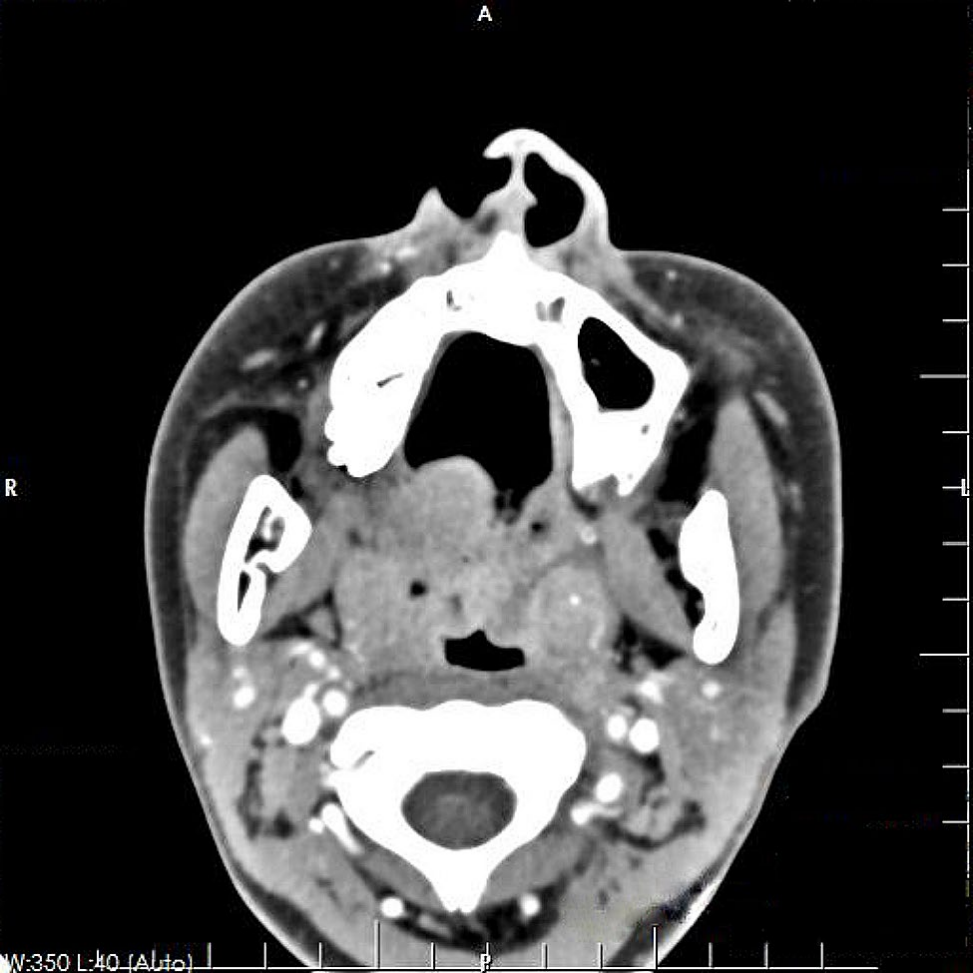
Contrast enhanced CT showed a slightly swollen uvula, bilateral tonsillar enlargement, and punctate calcification of the left tonsil
